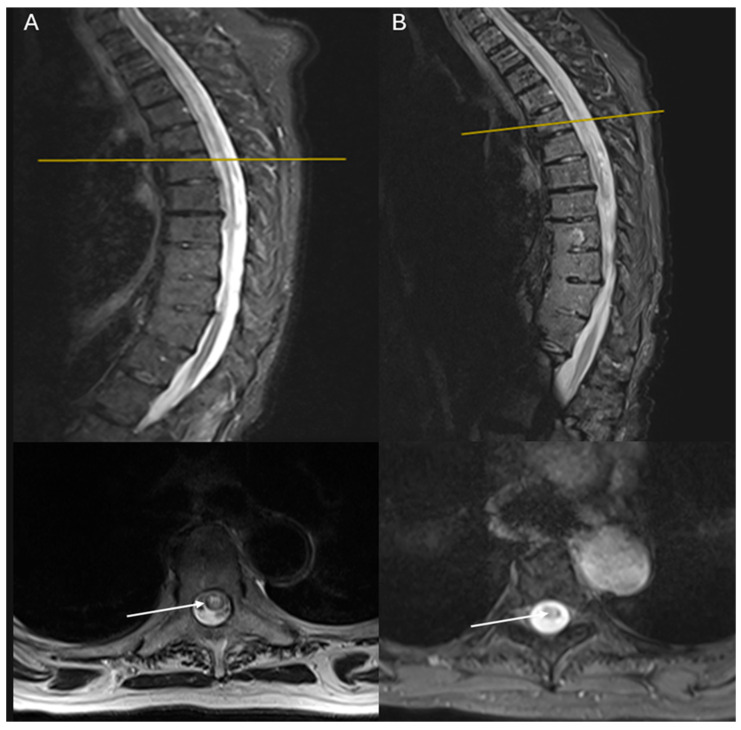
MRI imaging of the first case demonstrating a long segment of a well-defined lesion extending from T3 to T8, appearing low on T1 with no gadolinium enhancement and high on T2:(A) sagittal and axial T2 STIR-weighted MRI images of the thoracic spine at admission; (B) sagittal and axial T2 STIR-weighted MRI images of the thoracic spine 1 year of follow-up. White arrows show the lesions of hyperintensities involving the central and the right half of the cord, sparing the dorsolateral part. One year later, lesions had the same extent but weaker T2 hyperintensity.
